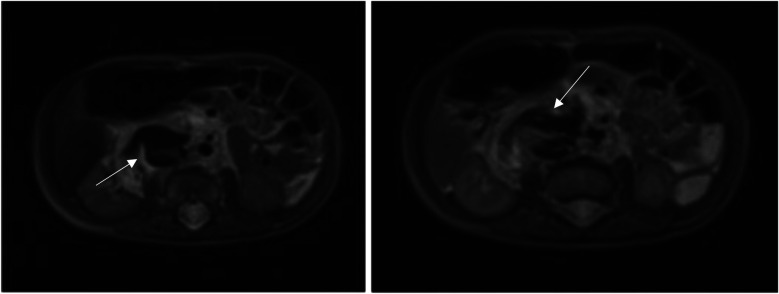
Intralesional arteriovenous shunt on axial section at MRI.
